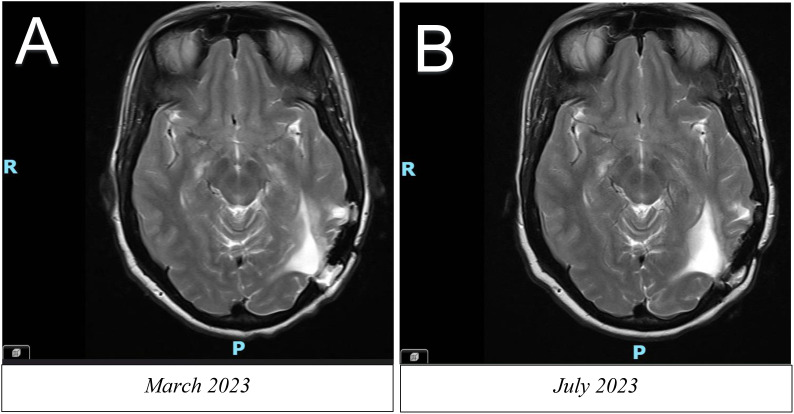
Brain MRI performed in March 2023 at the initiation of T-DM1 therapy (A) and after four months of TDM1 treatment (B).
